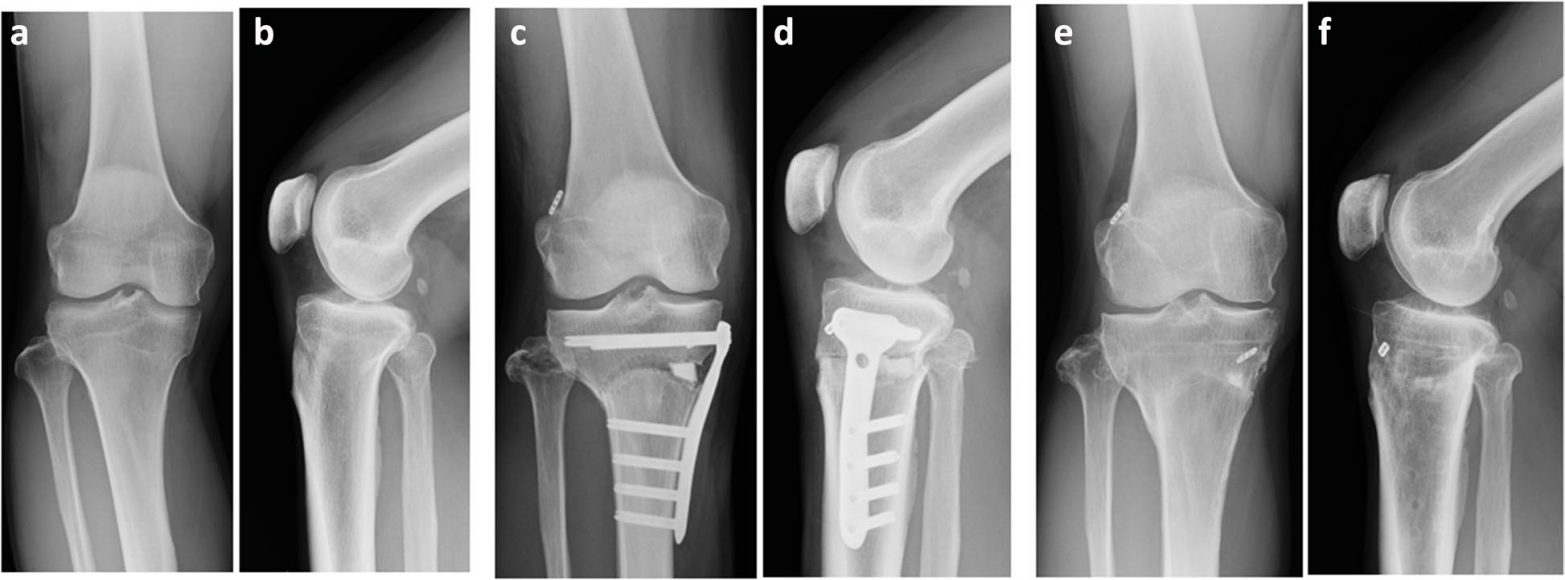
a Dome-shaped high tibial osteotomy combined with all-inside anterior cruciate ligament reconstruction using semitendinosus tendon simultaneously at pre and post operative radiography. a Standing antero-posterior view, b Lateral view of pre operation. b Standing antero-posterior view, c Lateral view of postoperative 3 weeks,. d Standing antero-posterior view, e Lateral view of postoperative 3 years
